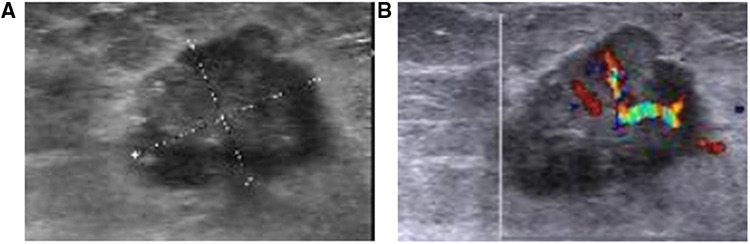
Breast sonography from a 42-year-old woman with a suspicious breast mass (A) the breast sonography shows a hypoechoic mass in the left breast, located 1 cm away from the nipple in the 6 O'clock direction, with a spiculated margin, irregular shape, and crab foot sign. (B) Color Doppler sonography shows the presence of large blood vessels in the tumor, with obvious arterial blood flow signals.
